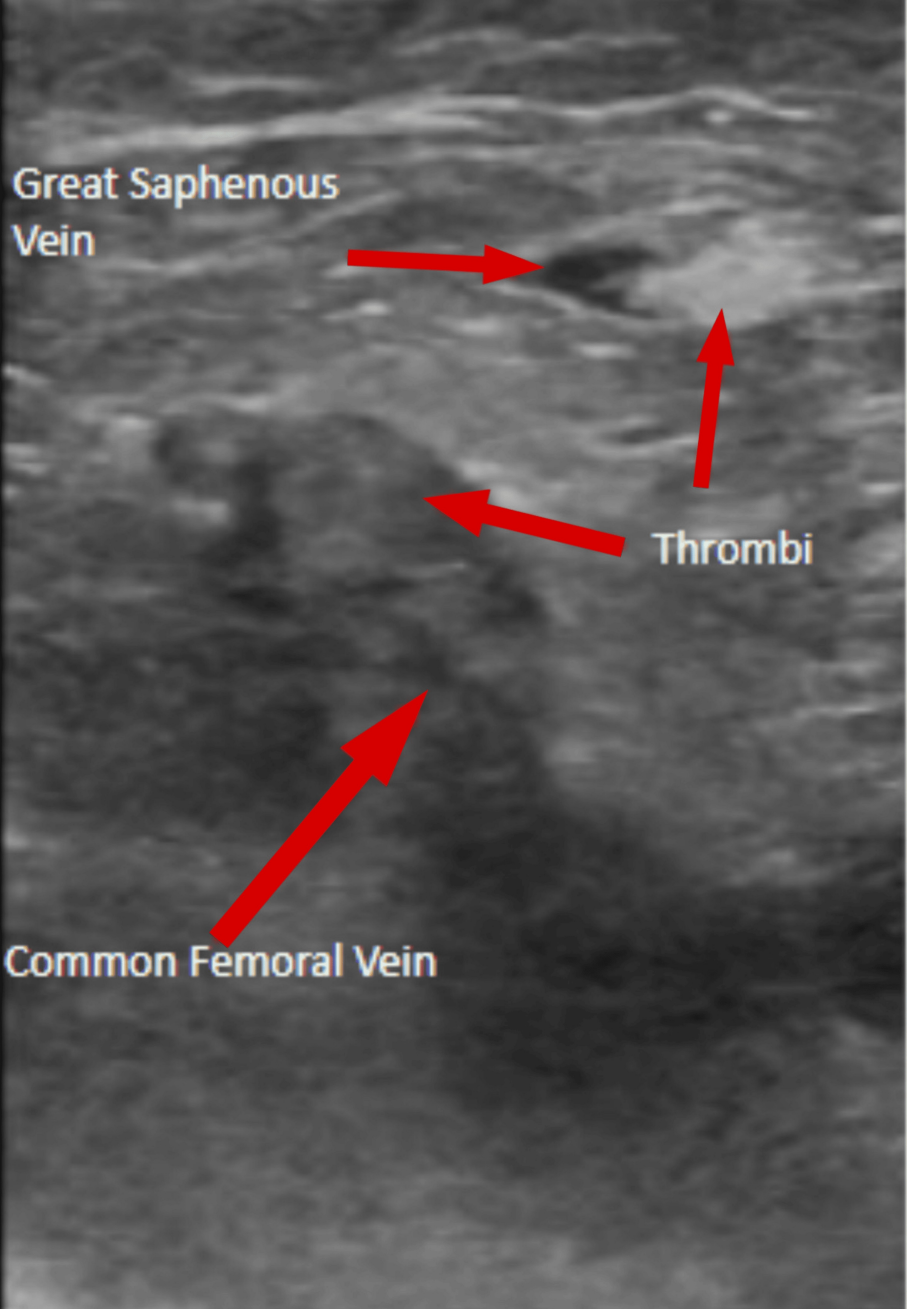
Ultrasound of the Left Lower Extremity Showing Thrombi
